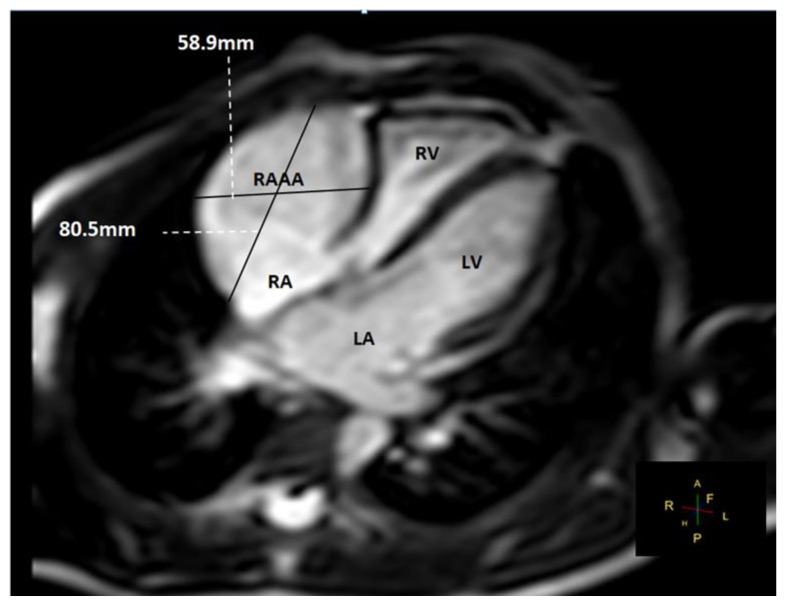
MRI in the same child eight years later. The initial gigantic aneurismal dilatation of the RAAA had diameters of 80 mm × 59 mm, reaching a total volume of 110 mL.
